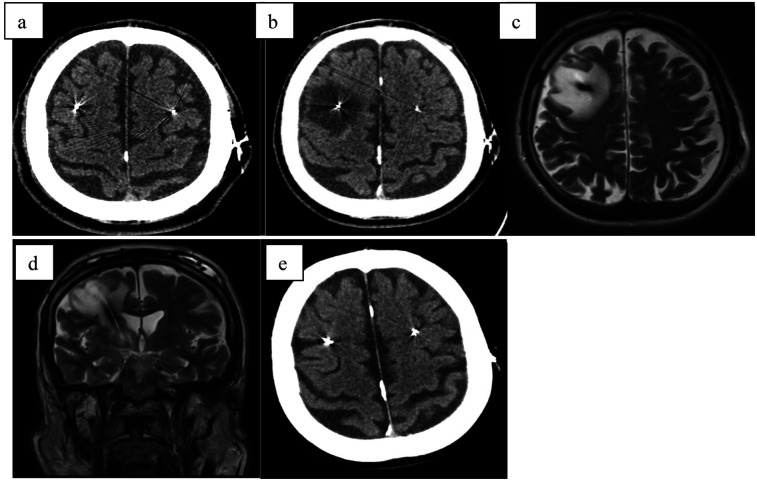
(a) CT scan directly after electrode implantation; (b) CT scan fourth day after surgery with hypodensity surrounding right electrode; (c) MRI-T2 transversal scan 1 week after surgery with large edema surrounding right electrode; (d) MRI-T2 coronar scan 1 week after surgery with large edema surrounding right electrode; (e) CT scan 3 months after surgery.
